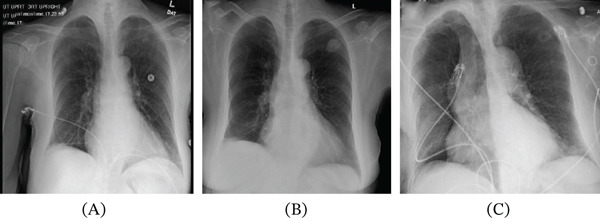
Chest radiographs demonstrating the evolution of the pulmonary lesion. (A) Chest radiograph obtained approximately 2 years prior, demonstrating a subtle right upper lobe opacity. (B) Chest radiograph obtained at presentation demonstrating a more prominent right upper lobe lesion. (C) Follow‐up chest radiograph at approximately 6 weeks demonstrating an interval decrease in lesion size and density, consistent with a resolving infectious process.
